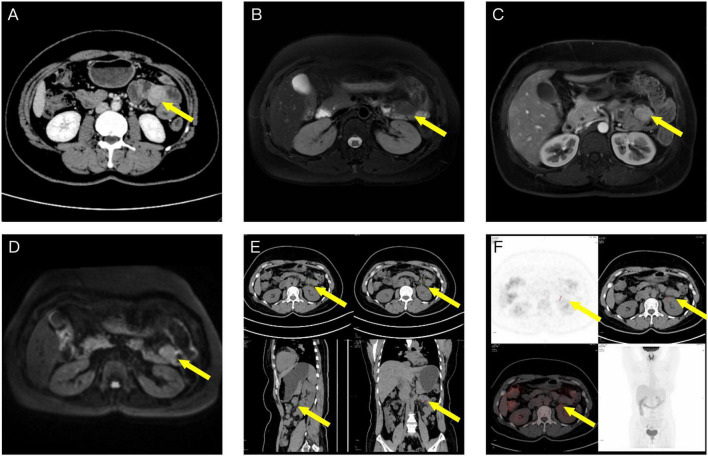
Preoperative imaging findings in Case 2. (A) Contrast-enhanced computed tomography (CT) revealed a nodular slightly hyperdense lesion on the mesenteric side of the proximal jejunum in the left mid-abdomen, measuring approximately 2.7 × 2.4 cm, with heterogeneous enhancement and an indistinct boundary from the adjacent bowel wall. (B) Fat-suppressed T2-weighted imaging (T2WI-FS) showed a slightly hyperintense lesion in the proximal jejunum. (C) Contrast-enhanced T1-weighted imaging (CE-T1WI) demonstrated enhancement of the lesion. (D) Diffusion-weighted imaging (DWI) showed mildly increased signal intensity in part of the lesion. (E,F) Positron emission tomography/computed tomography (PET/CT) demonstrated a soft tissue density lesion on the mesenteric side of the proximal jejunum measuring approximately 1.9 × 1.7 cm, with mildly increased fluorodeoxyglucose uptake and a maximum standardized uptake value (SUVmax) of 2.6. Arrows indicate the lesions.
